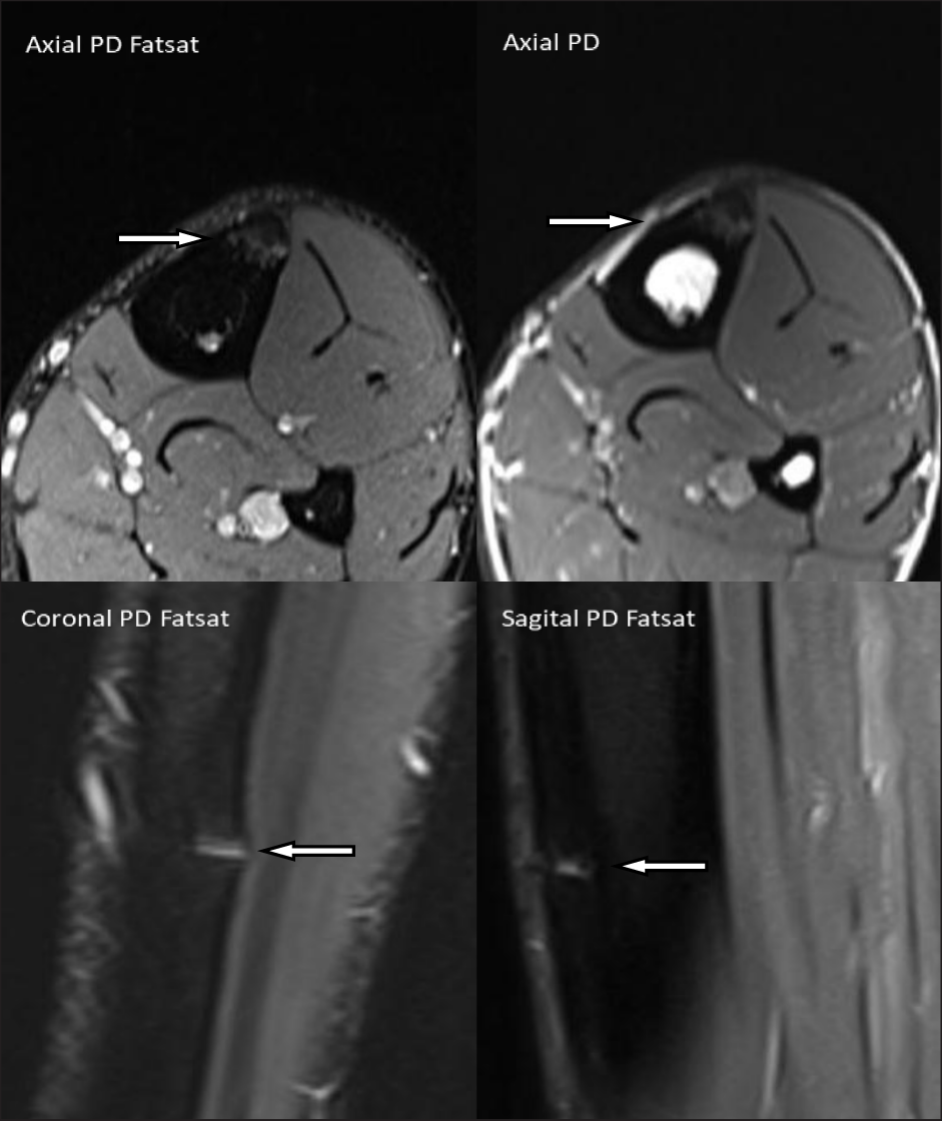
Additional X-ray (Figure 2) and computed tomography (CT) (Figure 3) examinations demonstrate multiple radiolucent lines in the anterior cortex of mid-tibial-diaphysis (dreaded black line sign) resulting from repeated episodes of stress fractures. The arrow indicates the current symptomatic fracture.
